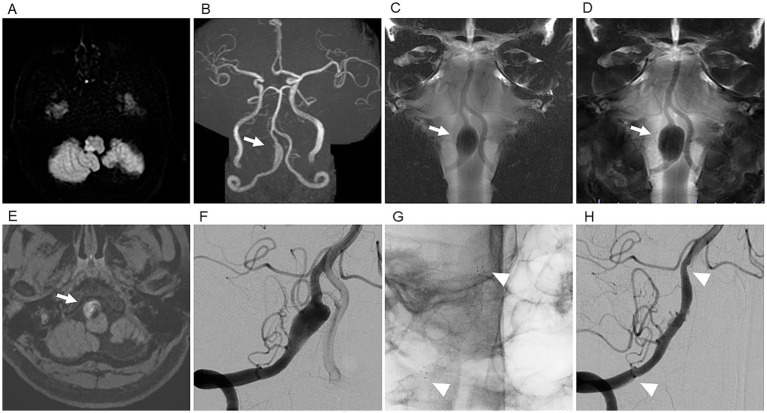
Representative case 2 (59-year-old man). (A) DWI on admission showing a right lateral medullary infarction. (B) TOF-MRA demonstrating right V4 dilatation. (C) BPAS showing outer-diameter enlargement, consistent with a partially thrombosed aneurysm. (D) BPAS obtained 7 years after initial onset showing enlargement of the aneurysm’s outer diameter. (E) 3D T1-weighted variable flip-angle TSE (T1-CUBE) image showing intraluminal thrombus (white arrow). (F) Preoperative DSA showing a dissecting aneurysm distal to the PICA origin. (G) Fluoroscopic image after FRED placement (arrowheads) across the V3–V4 junction. (H) Follow-up DSA 1 year after intervention demonstrating OKM C3 remodeling with maintained branch patency. White arrowheads indicate the distal and proximal edges of the flow diverter stent.
